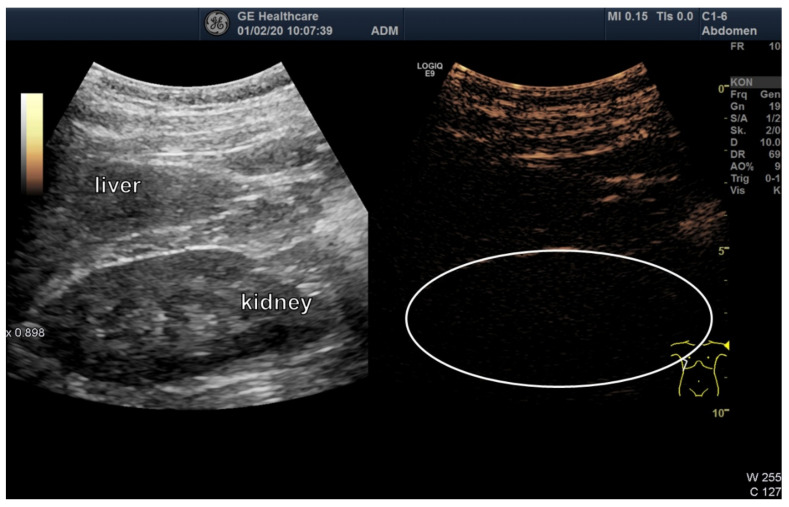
Ultrasound image of kidney. No enhanced signal is detected in the kidney.
